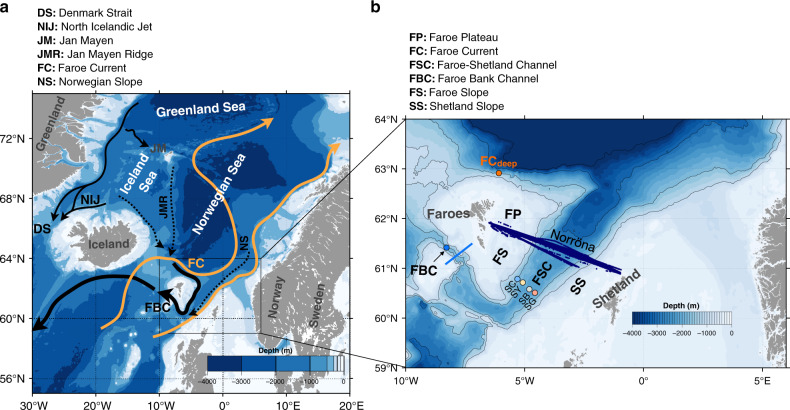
Nordic Seas overturning circulation pathways. a Bathymetric chart (shading; m) with the main upper-ocean (orange) and traditional deep (black) branches of the overturning circulation in the Nordic Seas. The dashed lines are deep branches suggested by earlier modeling studies to feed the Faroe Bank Channel^13,14^. b A zoomed map of the study region including locations of the multiple observational platforms used in this work. The circles refer to the deep Acoustic Doppler Current Profiler (ADCP) moorings (see Methods section). The light blue section is the hydrographic transect in the Faroe Bank Channel. The dark blue dots across the Faroe-Shetland Channel (FSC) indicate sites of velocity profiles from the vessel-mounted ADCP onboard MS Norröna. The black contours depict the 500, 800, 1500, and 2000 m isobaths.
